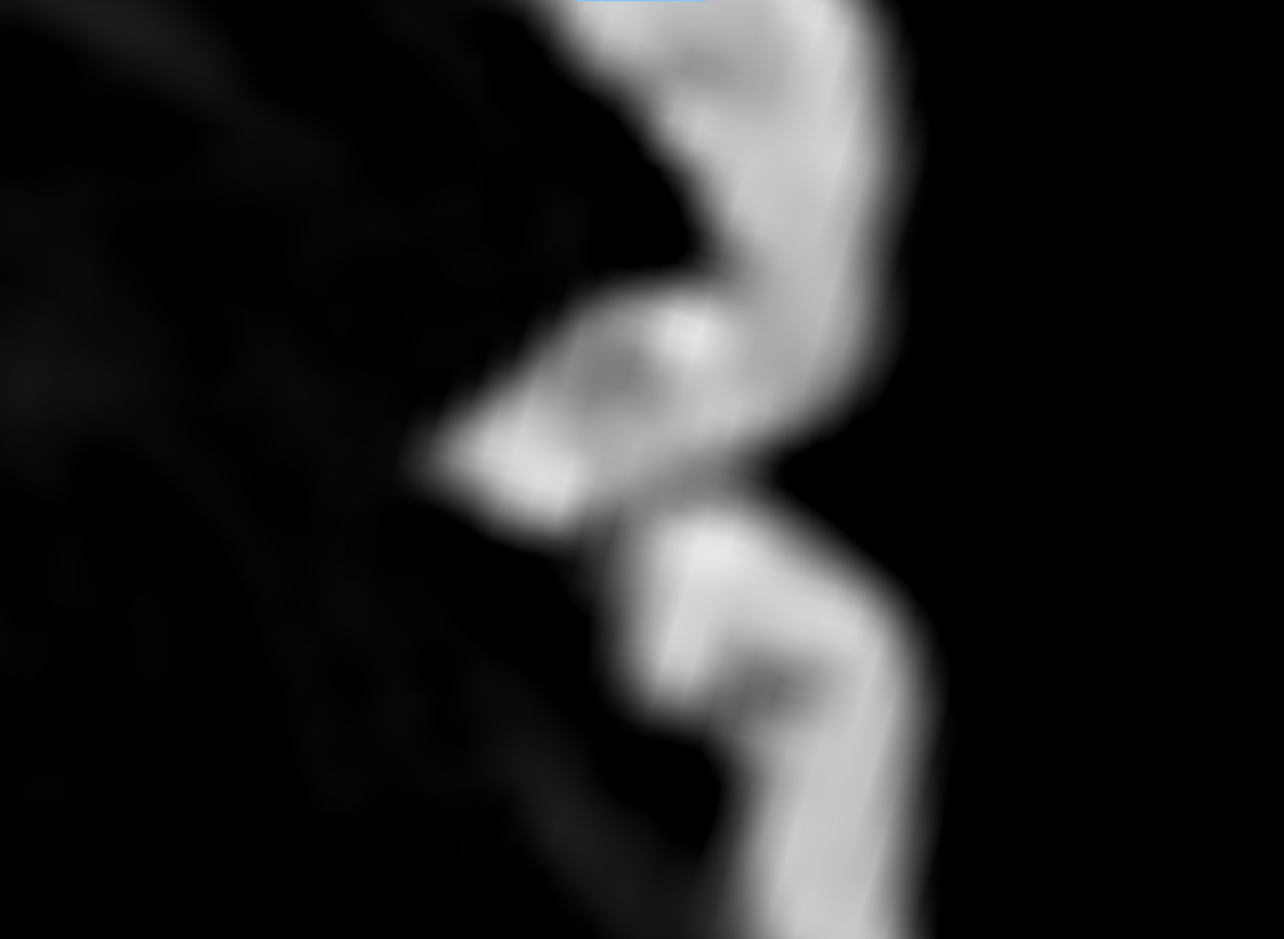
Comparative slice images of the eye at different resolutions. Clinical CT coronal slice through the eye.
